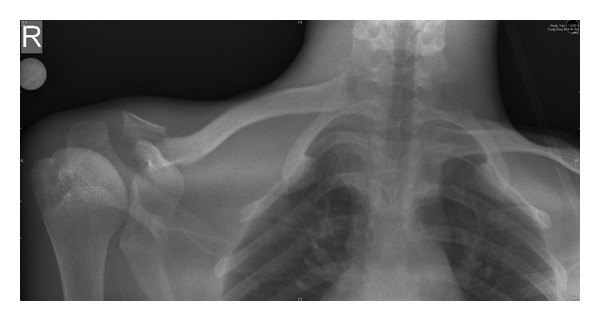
Radiograph at first presentation showing fracture lateral end of right clavicle and sternoclavicular dislocation—the floating clavicle.
